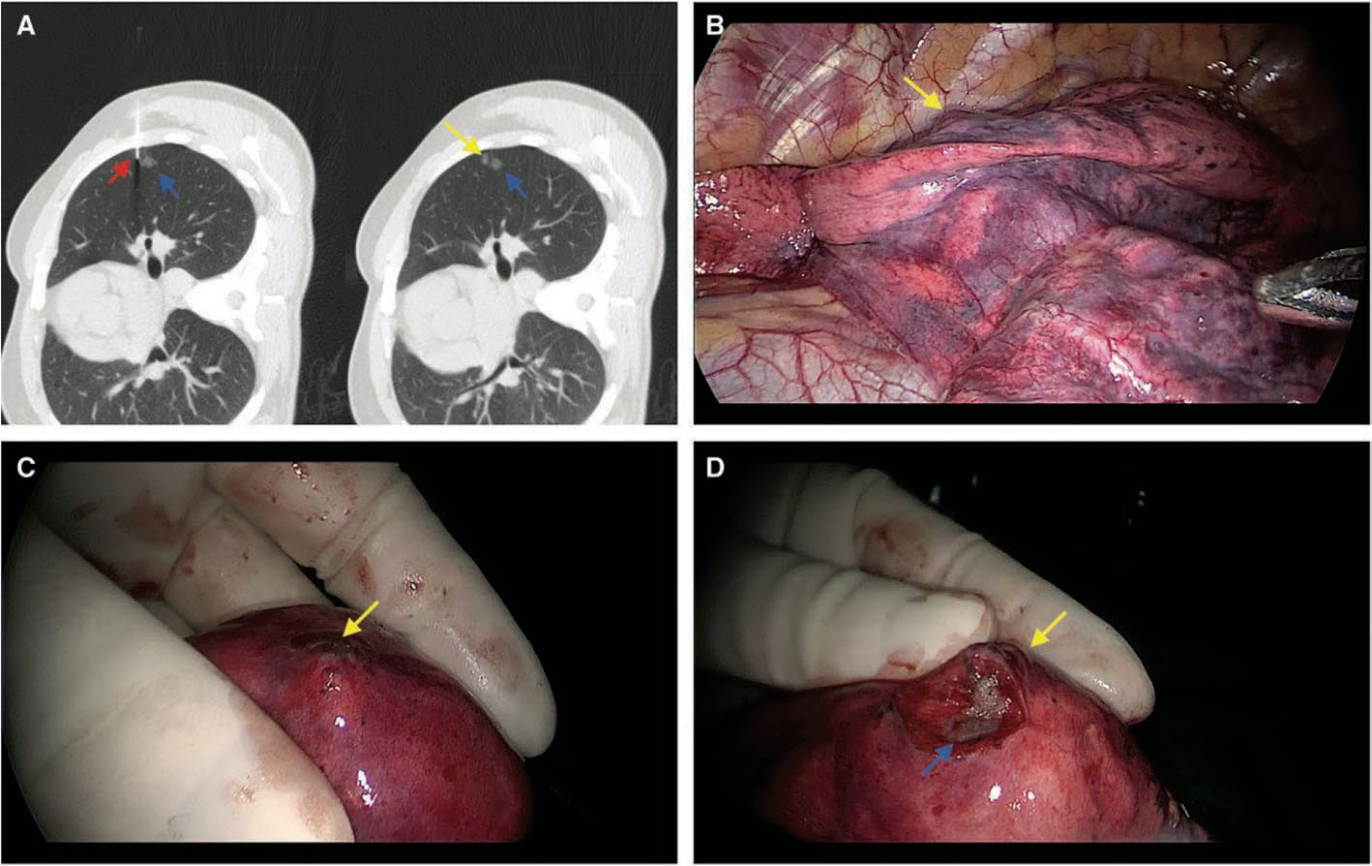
The CT-guided cyanoacrylate localization [6]
